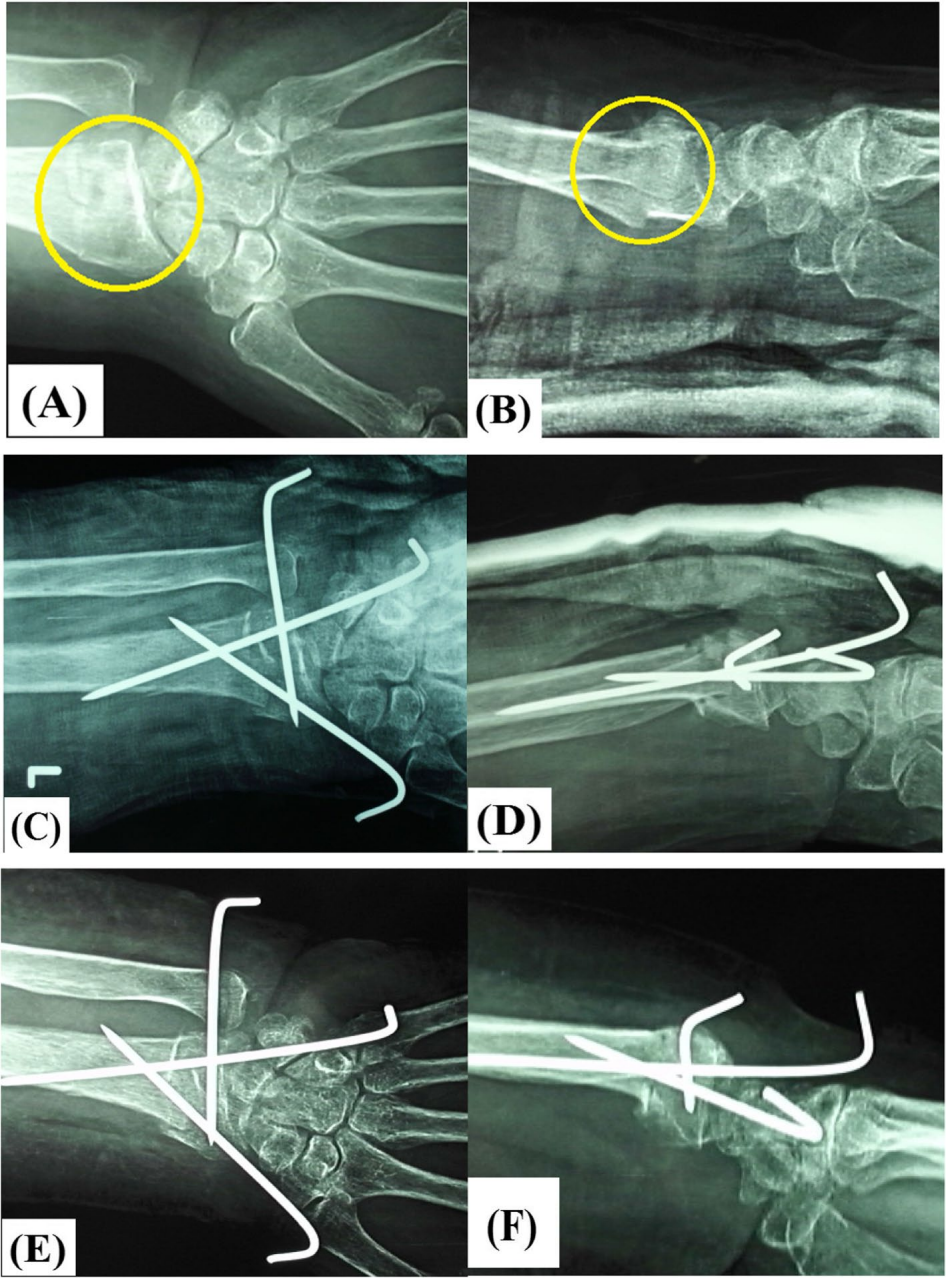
demonstrate insignificant secondary displacement of postoperative radiographic parameters of unstable intra-articular distal radius fracture in 64-year old female patient managed by stand-alone percutaneous pinning; A & B preoperative antero-posterior and lateral X-ray views of the fracture (marked in yellow circle); C & D immediate postoperative antero-posterior and lateral X-ray views of the fracture; E & F 6-week postoperative antero-posterior and lateral X-ray views of the fracture
